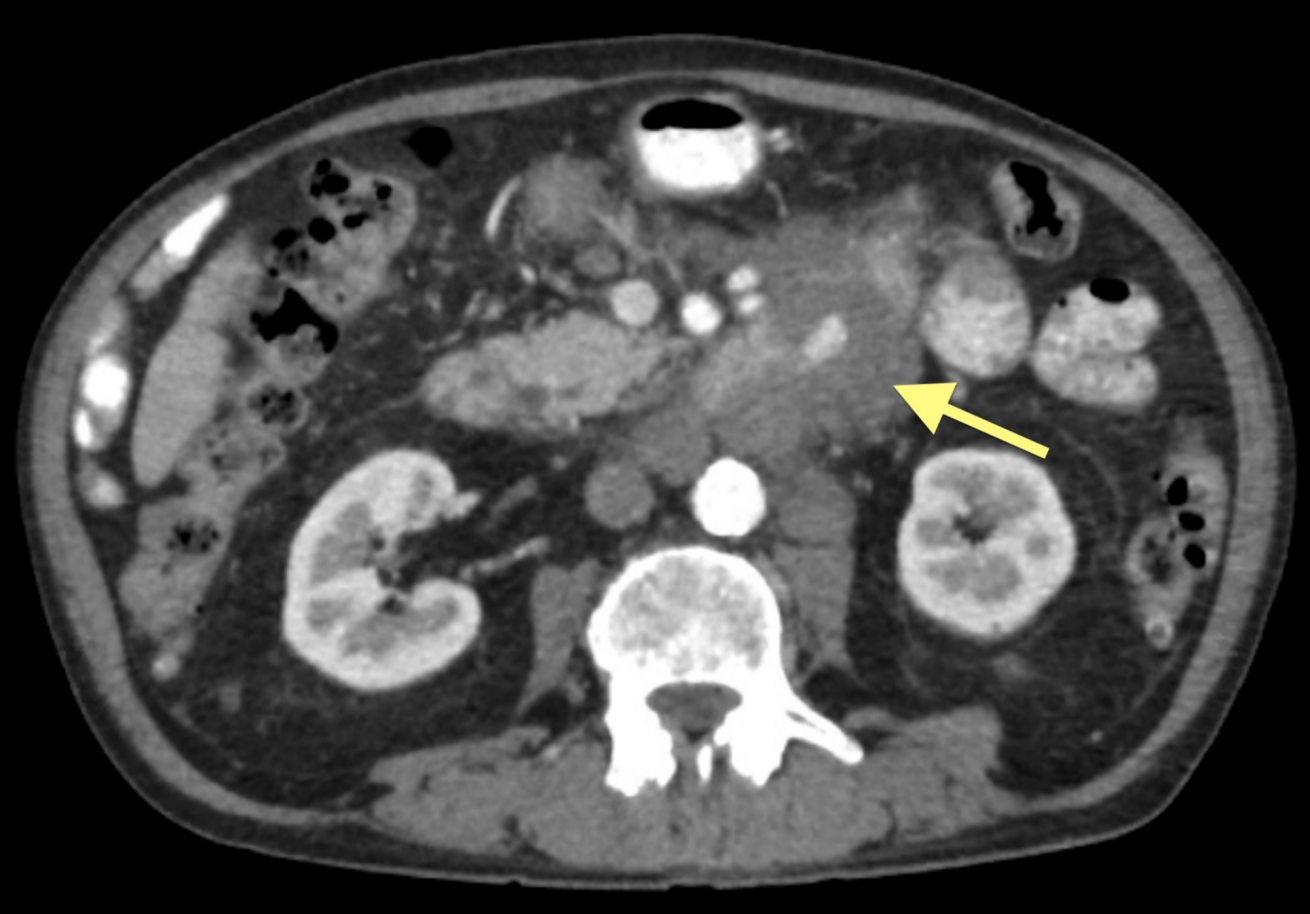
CT of abdomen and pelvis with contrast demonstrating duodenal angiosarcoma CT, computed tomography
